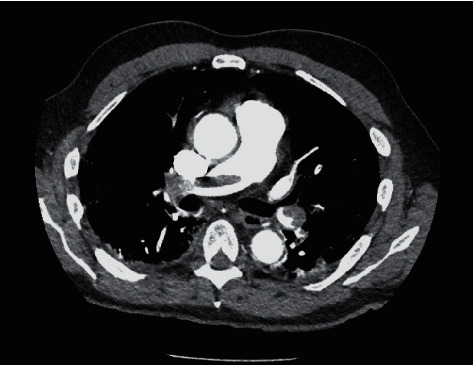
CT angiogram with saddle embolus.
